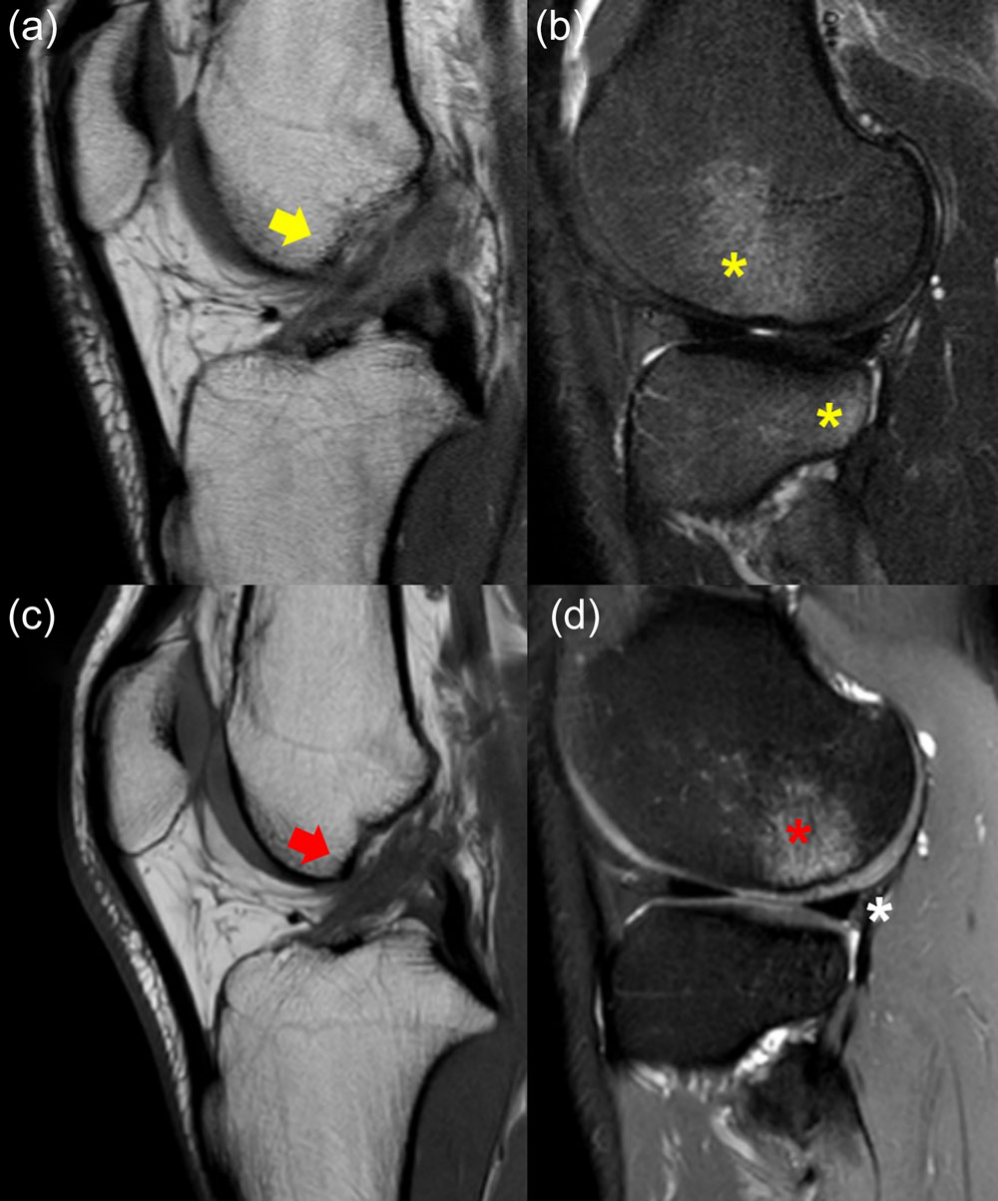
Left knee of a 22‐year‐old patient with an acute partial ACL tear. The sagittal MRI showed altered signal and continuity of the ACL (yellow arrow) (a) and the classic ACL‐injury‐related bone bruises of the anterior lateral femoral condyle and posterolateral tibial plateau (yellow asterisks) (b). After 4 years, at the age of 26, the sagittal MRI shows the altered signal of the ACL (red arrow) (c) and the presence of subchondral bone oedema (red asterisk) in the posterior portion of the lateral femoral condyle, with an intact lateral meniscus (white asterisk) (d). ACL, anterior cruciate ligament; MRI, magnetic resonance imaging.
